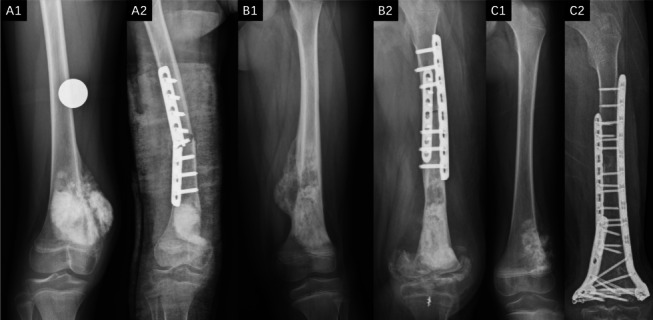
Representative cases of different internal fixation strengths in tumor bone inactivation and replantation surgery. A1 is preoperative X-rays of a patient with osteosarcoma in the right femur, and A2 shows the X-ray of this patient’s fracture 4 months after surgery. B1 is preoperative X-rays of a patient with osteosarcoma in the left femur, and B2 shows the X-ray of this patient's fracture 12 months after surgery. C1 is preoperative X-rays of a patient with osteosarcoma in the left femur, and B2 shows the X-ray of this patient’s fracture 20 months after surgery
